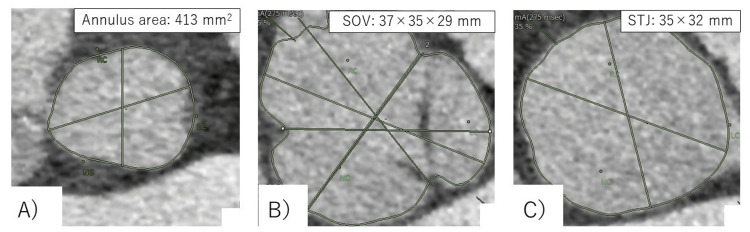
Preoperative CT around the aortic valve A:  Annulus, B: Valsalva, C: Sinotubular junction The CT revealed that the patient's anatomy was compatible with the 23 mm size of the Sapien 3 Ultra RESILIA (Edwards Lifesciences, Irvine, CA, USA). There was virtually no calcification of the aortic annulus or cusp. SOV: Sinus of Valsalva, STJ: Sinotubular junction
